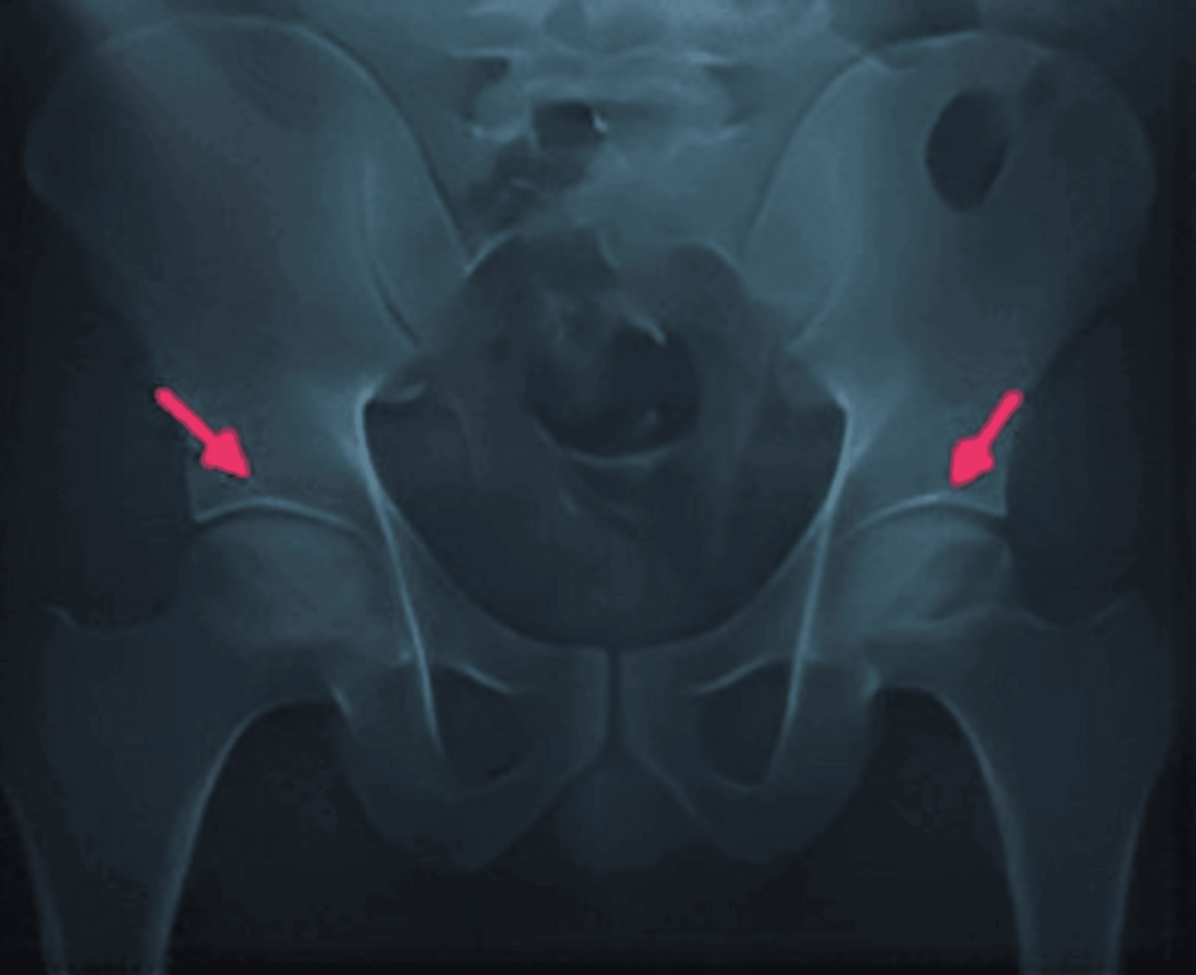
X-ray of the pelvis and both hips of case 3, AP view. Findings: Flattening, cortical collapse, and sclerosis of the bilateral femoral head, representing bilateral Ficat and Arlet stage III AVN (red arrow). AP, anteroposterior; AVN, avascular necrosis
